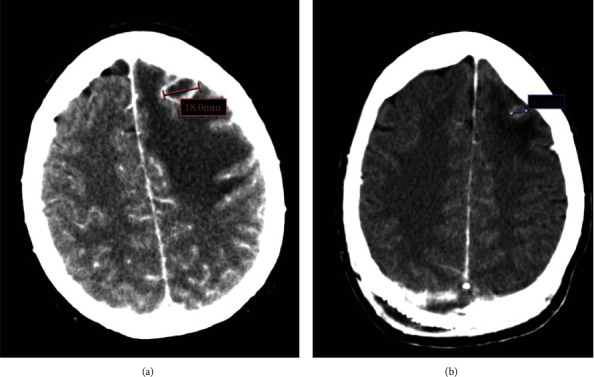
Image of left frontal lobe lesion from CT scan at diagnosis (a) and reevaluation CT scan performed six weeks after the initiation of osimertinib (b), showing a partial response (from 18 mm to 8 mm).
